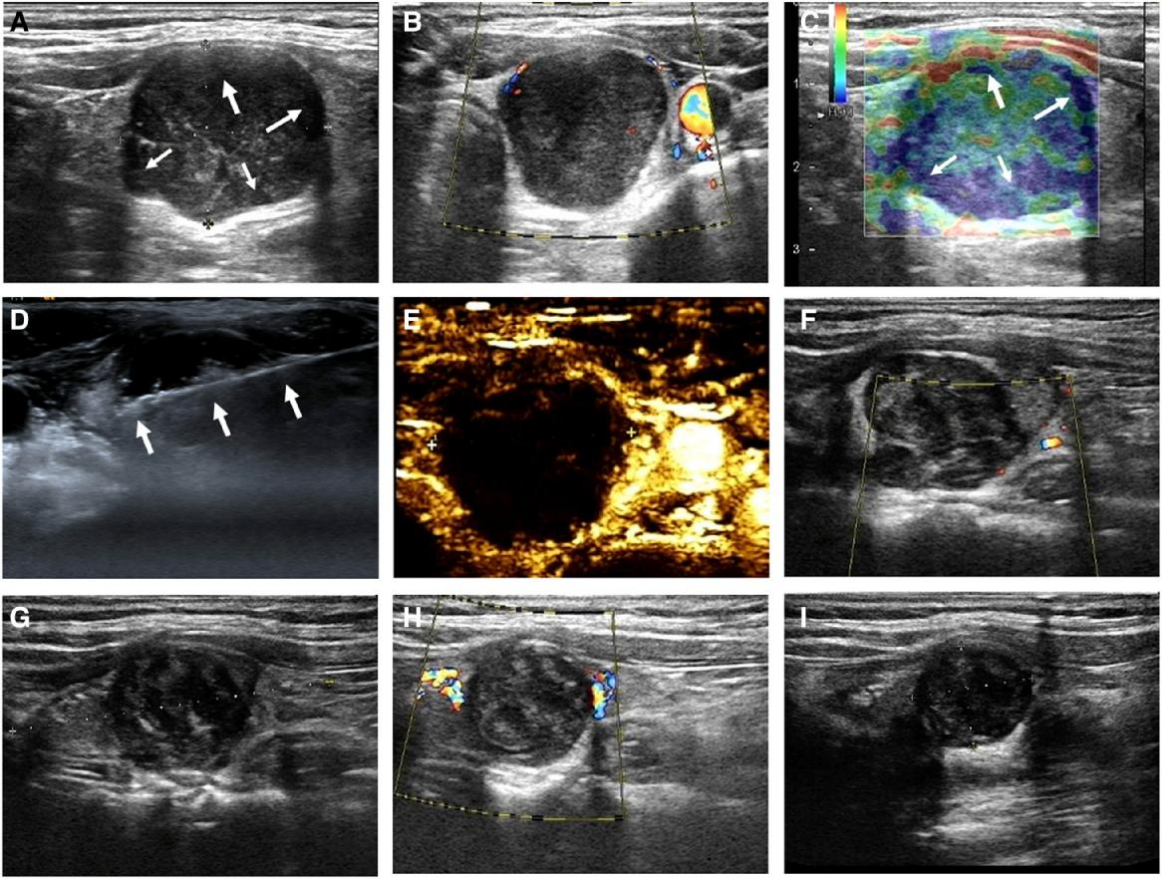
Representative preoperative, intraoperative, and postoperative ultrasound scans of the thyroid. A, Preoperative gray-scale ultrasonography of the thyroid showing a heterogeneous hypoechoic nodule in the left lobe. B, Color Doppler ultrasound of the thyroid indicating less vascularity at the margin of the thyroid nodule. C, Elastography showing the target sign (white arrows) of the thyroid nodule with central soft tissues (green) and surrounding hard tissues (blue). D, Intraoperative ultrasonography depicting the microwave antenna (white arrows) inserted into the thyroid nodule. E, Ultrasonography the day after microwave ablation showing the absence of perfusion in the ablation zone. F to I, Ultrasonography at F, 1; G, 3; and I, 12 months postoperatively demonstrating a gradual reduction in tumor volume.
